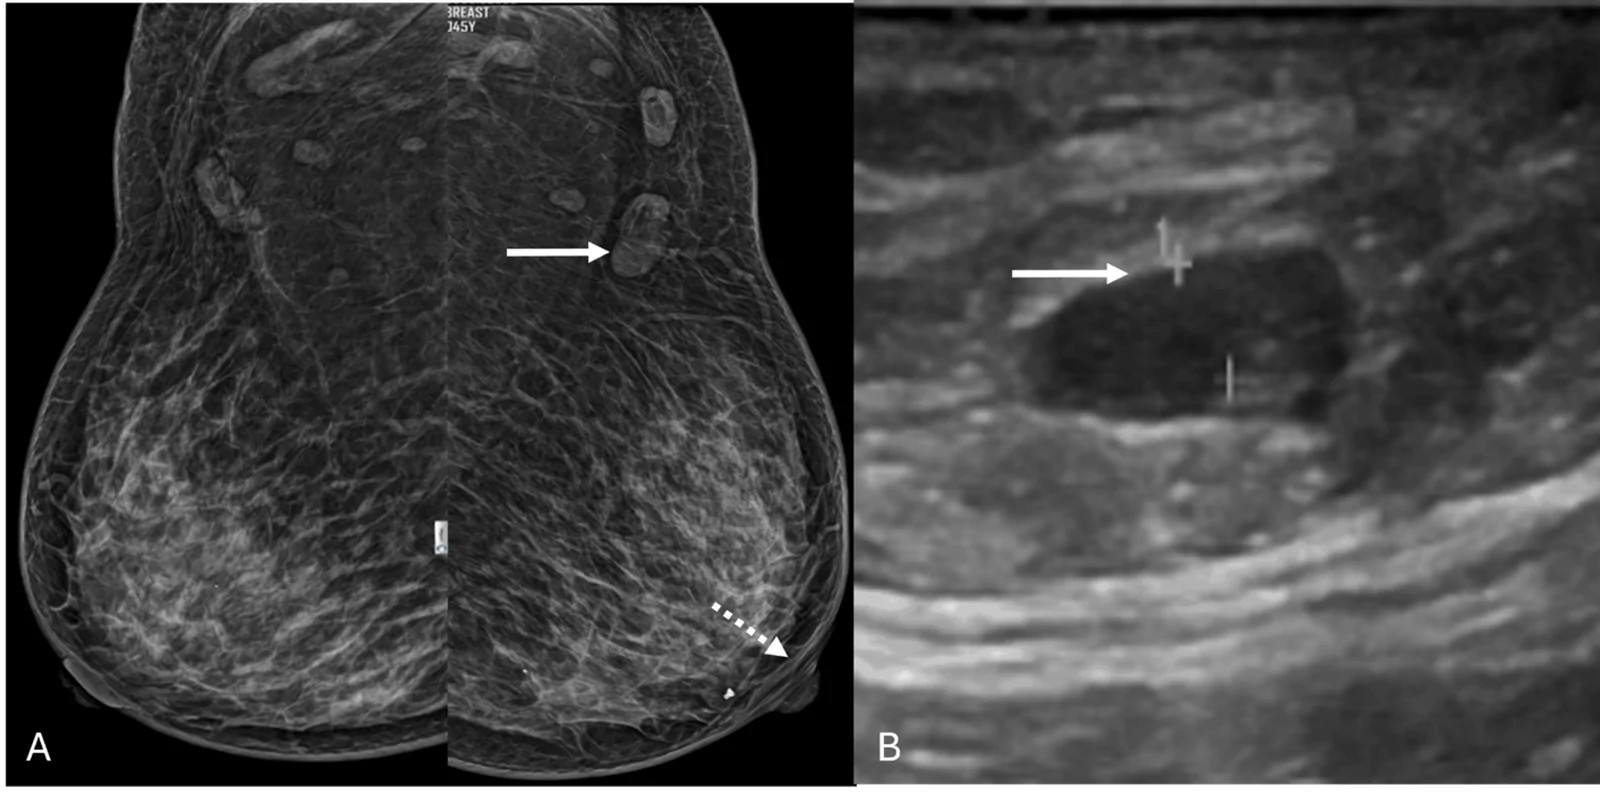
Mammographic and ultrasonographic findings demonstrating retroareolar skin thickening and abnormal left axillary lymphadenopathy MLO: mediolateral oblique (mammographic view) (A) Mammogram-MLO view of both breasts showing an enlarged left axillary lymph node (solid arrow) with skin thickening in the retroareolar region as compared to the right side (dotted arrow). (B) Ultrasonogram showing a level 1 left axillary abnormal lymph node with nonuniformly thickened cortex (solid arrow)
